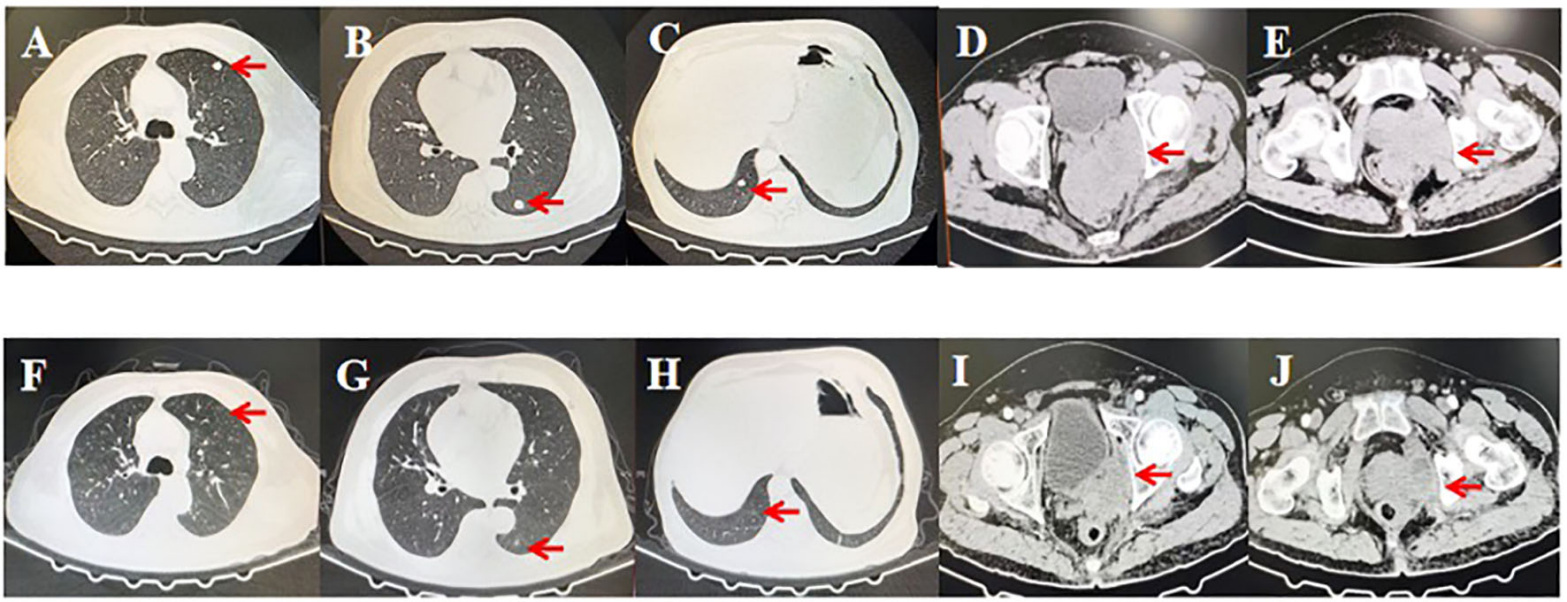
Computed tomography scans before and after treatment with nivolumab alternating with ipilimumab and doxorubicin liposomes. (A-E) Lung involvement and primary lesions of the prostate before initiating treatment. (F-J) Substantial reduction in lesions after completing six cycles of treatment. Red arrows indicate the lesions.
